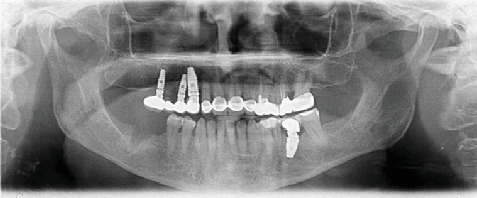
Panoramic radiograph at the 5-year follow-up.
